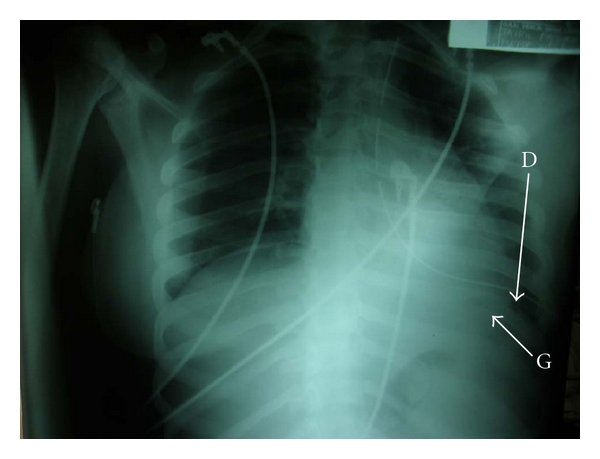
Immediate post-operative chest X-ray demonstrating a well formed left diaphragmatic outline (D), cardiac silhouette and an intra-abdominal gastric gas shadow (G).
